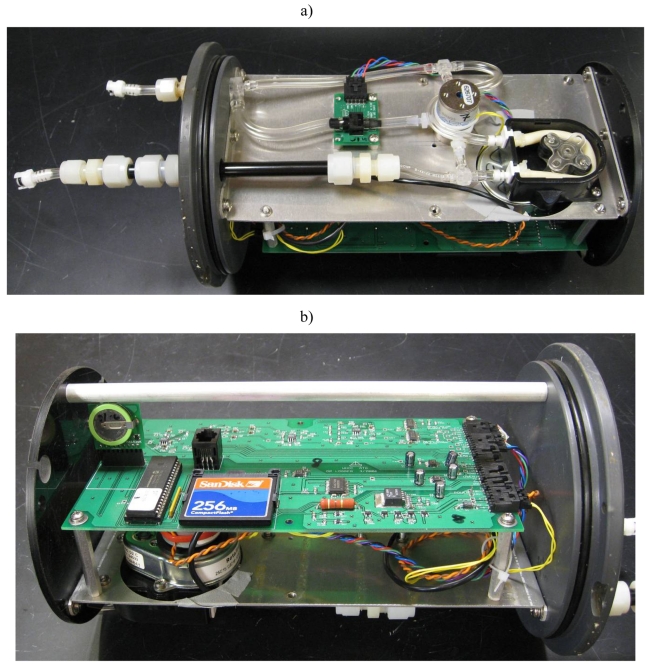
a) The internal fluid system mounted to the chassis – clockwise from upper left: groundwater port, DP cell with driving circuit board, solenoid valve, peristaltic pump, 545 CT cell feeding through the end cap to the surface water port; b) The motherboard of the logger mounted to the instrumentation chassis. The small vertical circuit board on the far left is the real-time clock. Next to the clock is the PIC processor, then the Compact Flash card. Beneath the processor, the motor for the peristaltic pump can be seen. The connectors on the right side of the board handle all interconnections to batteries, sensors, fluid components, and the user serial port.
